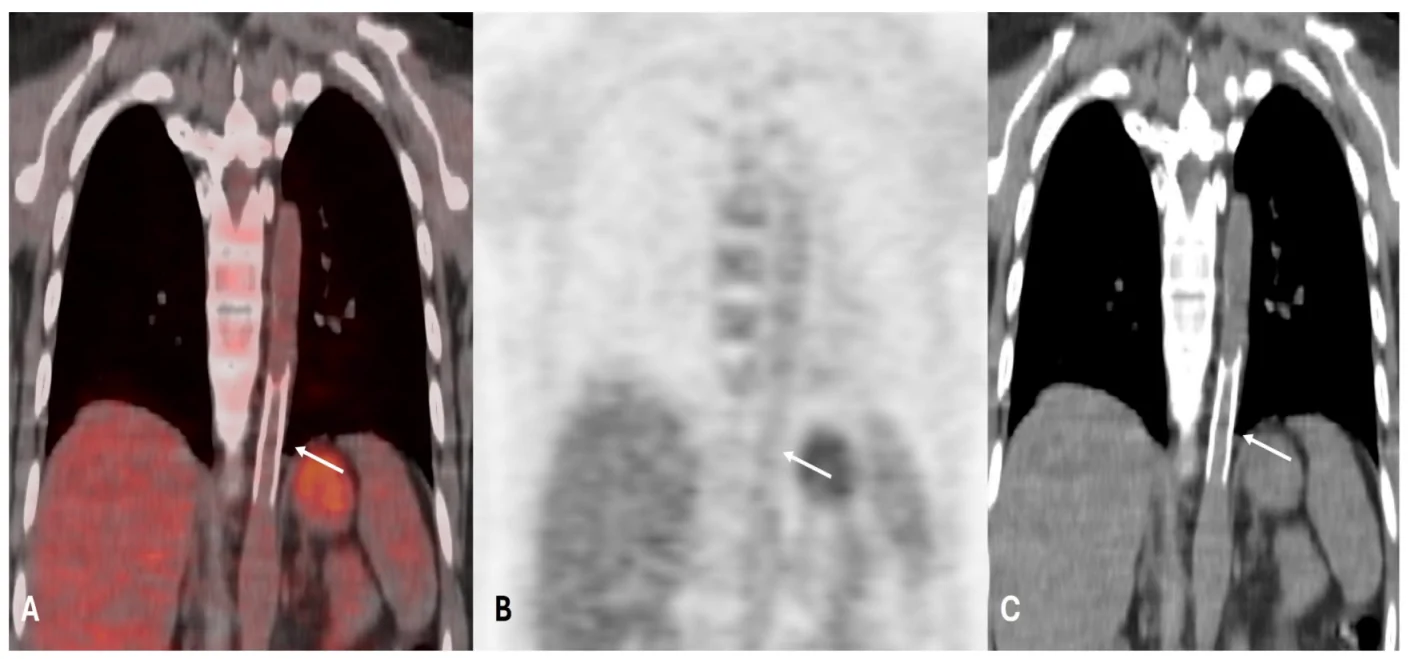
An example of known Takayasu’s disease status post descending aorta stent placement on remission. Coronal images of F-18 FDG PET/CT (A) fused, (B) AC PET and (C) CT without contrast showing a distal descending aorta stent with residual mild luminal narrowing (arrows). There is normal radiotracer distribution with no pathological aortic wall activity to suggest active inflammation.
